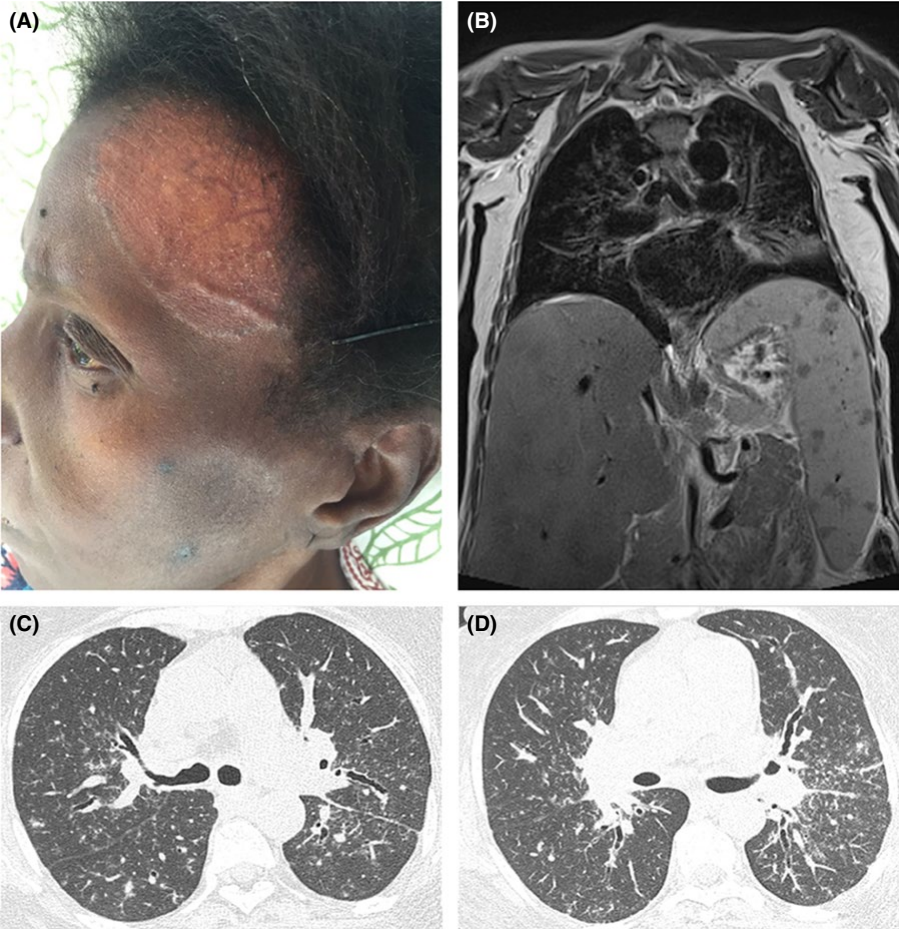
Ectoscopic examination showed a large, flat‐topped skin lesion on the forehead (A). Thoracoabdominal MRI displays hepatosplenomegaly with multiple splenic nodules, hypointense on a coronal T1‐weighted fat‐suppressed image (B). Peribronchovascular interstitial thickening was also identified. Chest CT demonstrates multiple small nodules predominating along the bronchovascular bundles (C, D). Bilateral hilar enlargement was also detected
